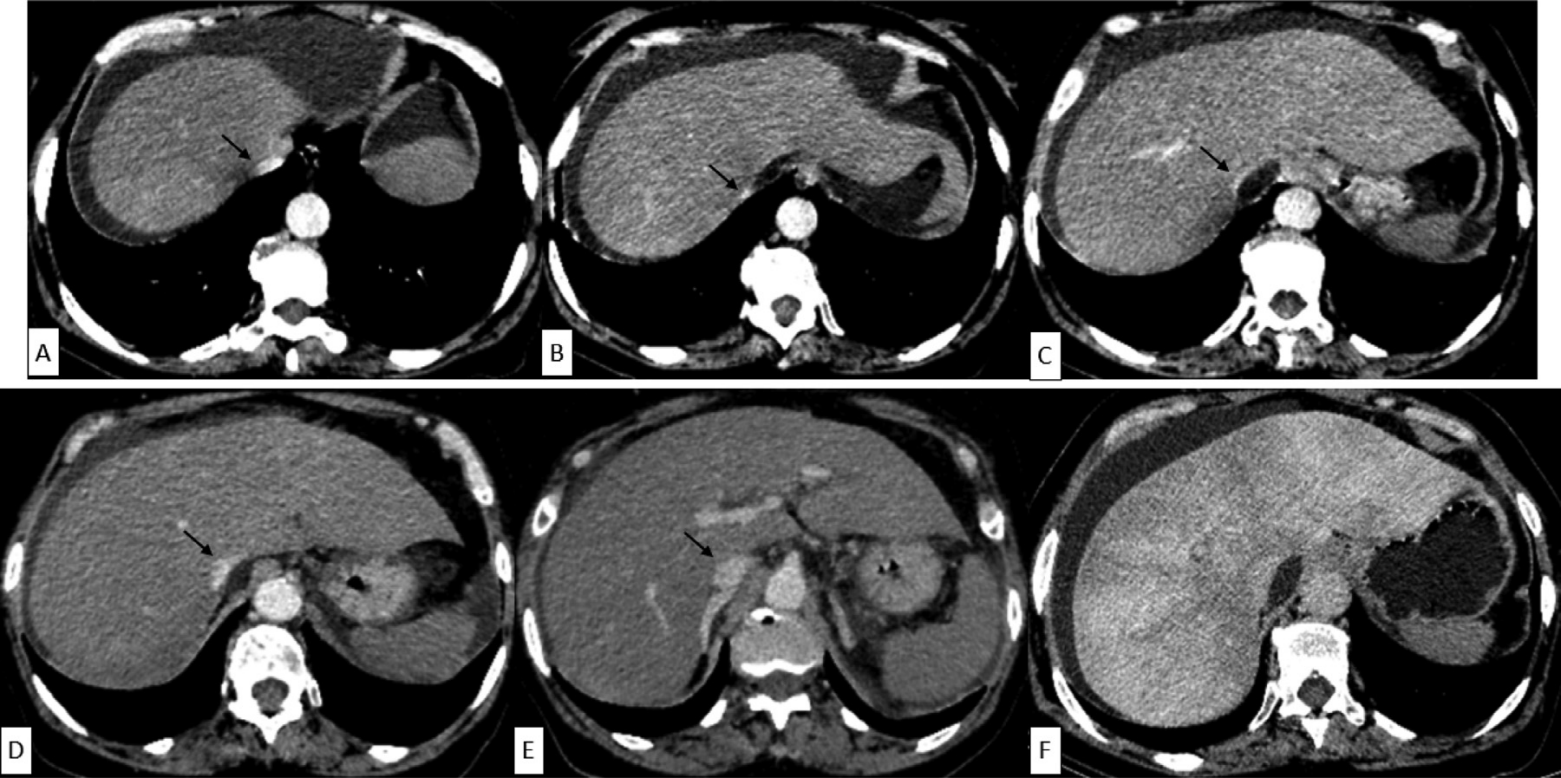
(A-F) Abdominal CT scan showing hypertrophy of right liver (C-F) with heterogeneous liver enhancement at the portal and delayed phases (F), without visualization of supra-hepatic main veins and retracted appearance of retro-hepatic vena cava (black arrows) which still minimally opacified at the periphery (B,C). The supra-hepatic and the sub-hepatic inferior vena cava were well opacified (A,E).
